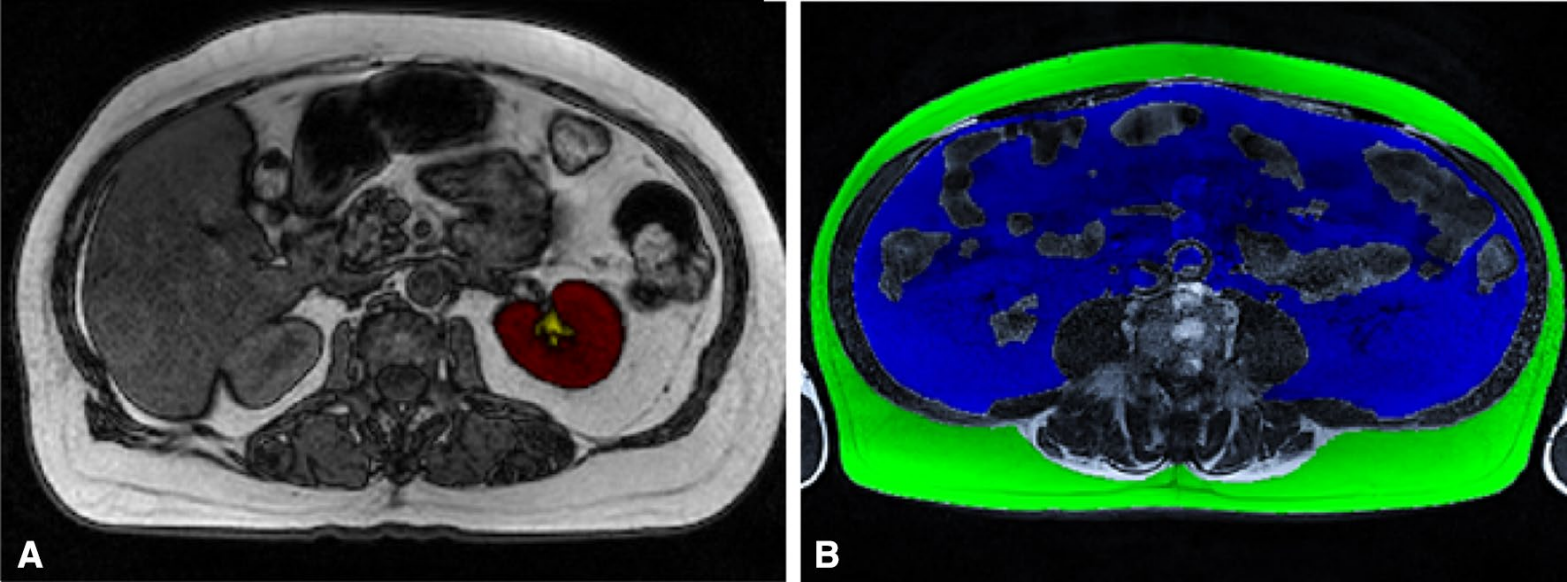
a Single MRI slice of RSF measurement. The total area of the left kidney (TK, red) and RSF (yellow) were manually traced and calculated. The renal artery and vein were traced to ensure they were excluded from the RSF measurements. b MRI scan of SAT (green) and VAT (blue) measurement
